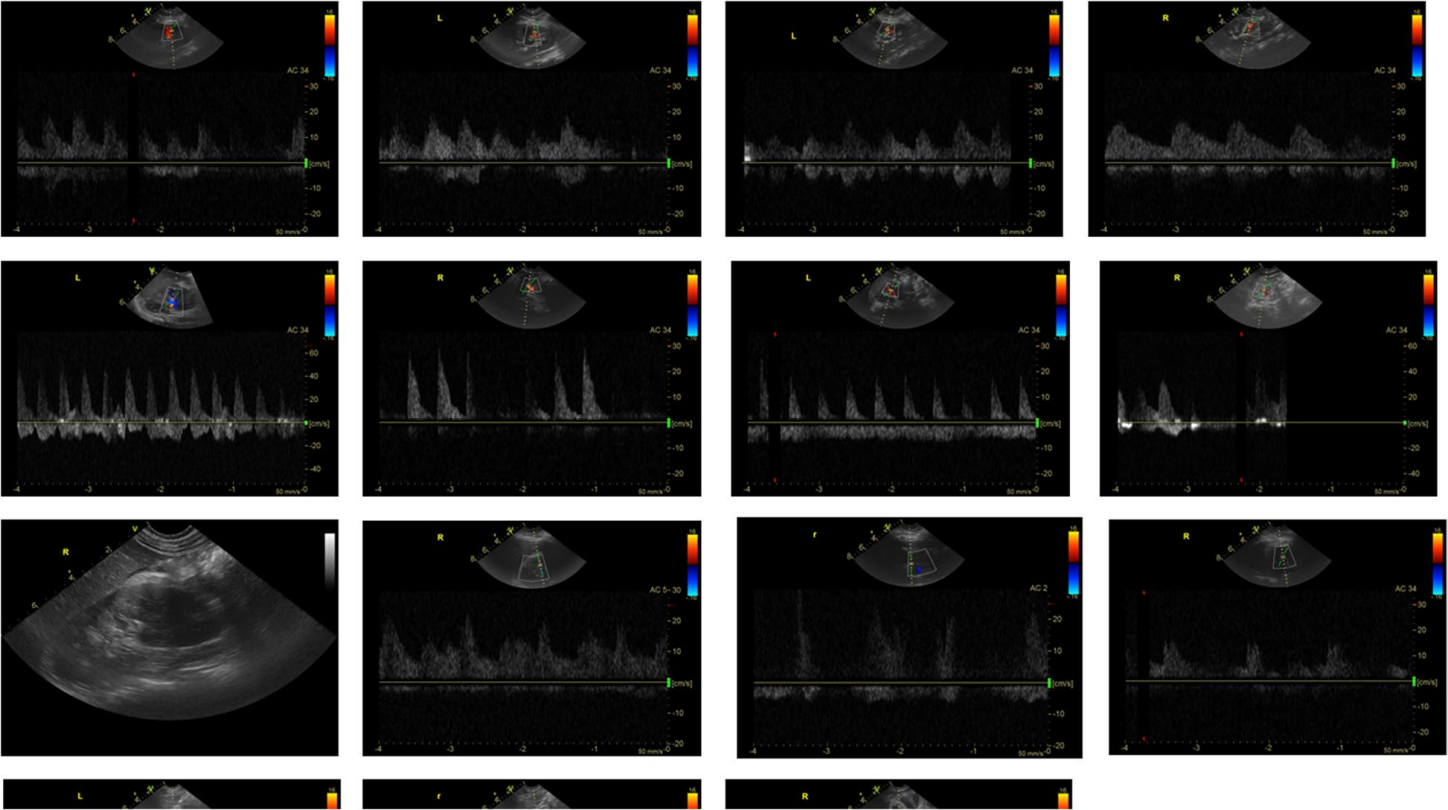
Dynamic ultrasound changes of shock patients. Note: From top to bottom, each line represents a patient, and from left to right each line represents the ultrasound changes of the patient from admission to the fourth day respectively
